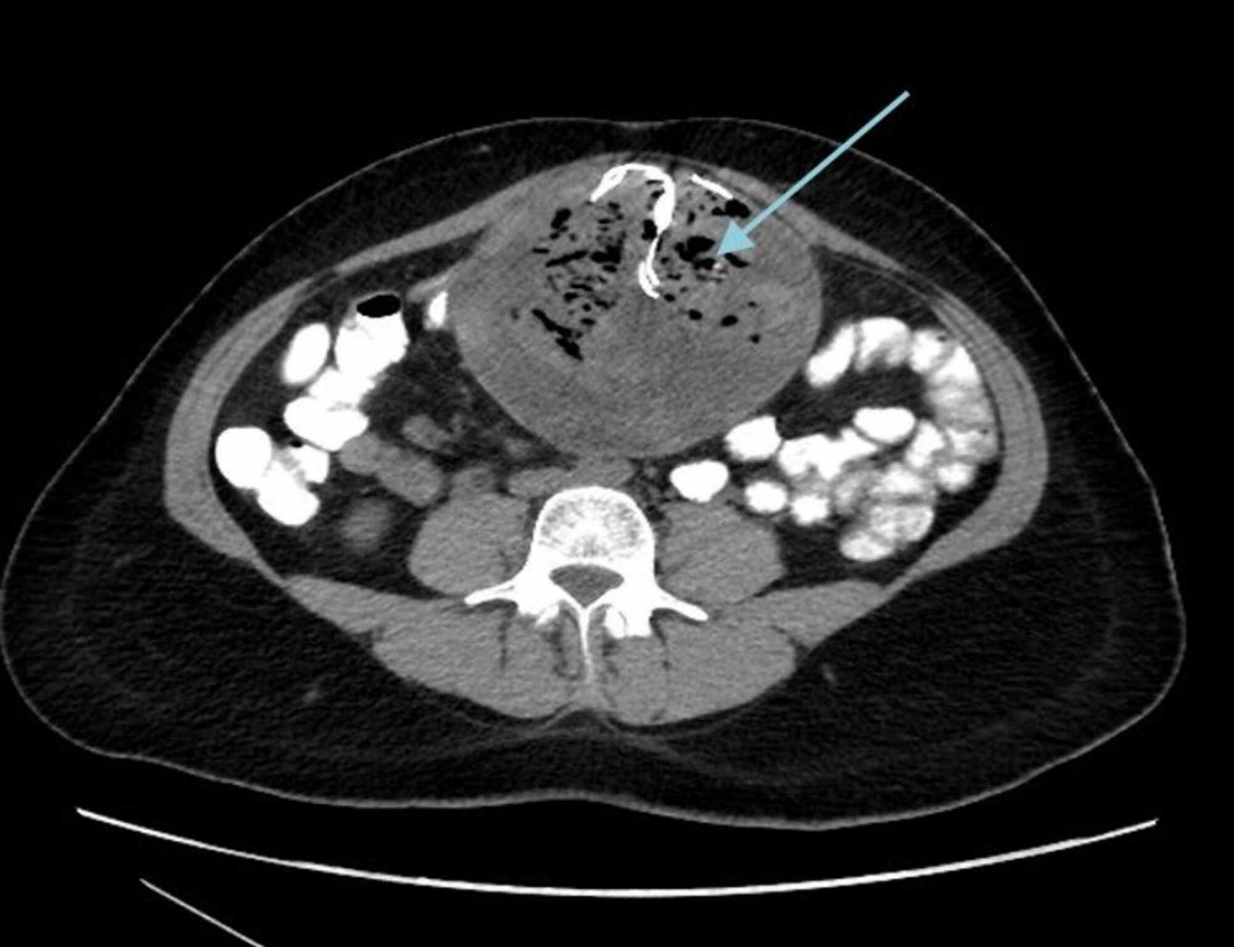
CT Abdomen showing intra-abdominal mass congaing air bubbles and hyperdense linear structure.
